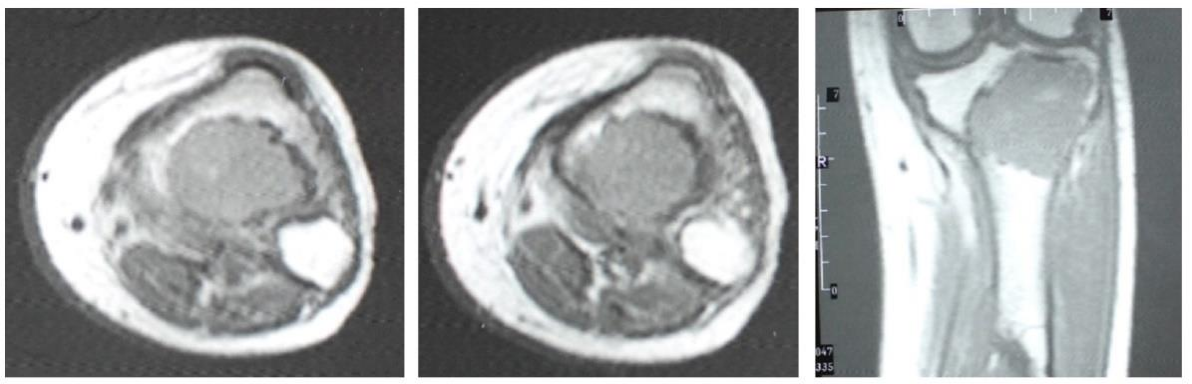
T1W Magnetic Resonance images show low signal expansile bony lesion with subarticular extension
